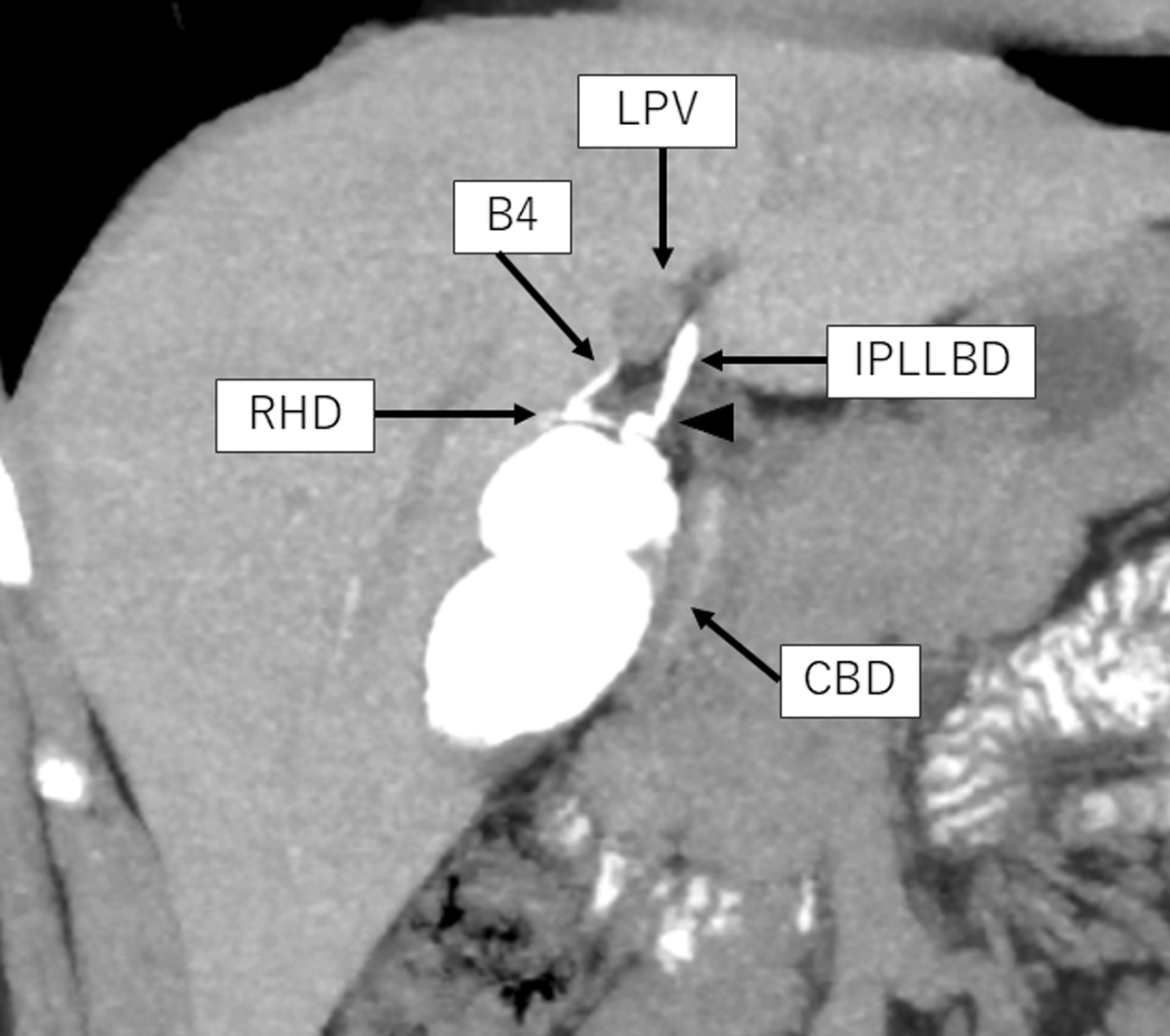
Computed tomography with drip infusion cholangiography revealed that the left lateral bile duct ran caudal to the umbilical portion, and this bile duct was defined as the infraportal type of the left lateral bile duct (IPLLBD). The left medial bile duct (B4) joins the right hepatic duct (RHD). The black arrowhead represents the confluence of the cystic duct and the IPLLBD. CBD, common bile duct; LPV, left portal vein.
